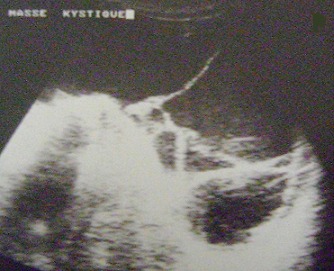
Ultrasonographic examination demonstrated a giant abdominal cystic lesion with internal echogenic particles and septations
